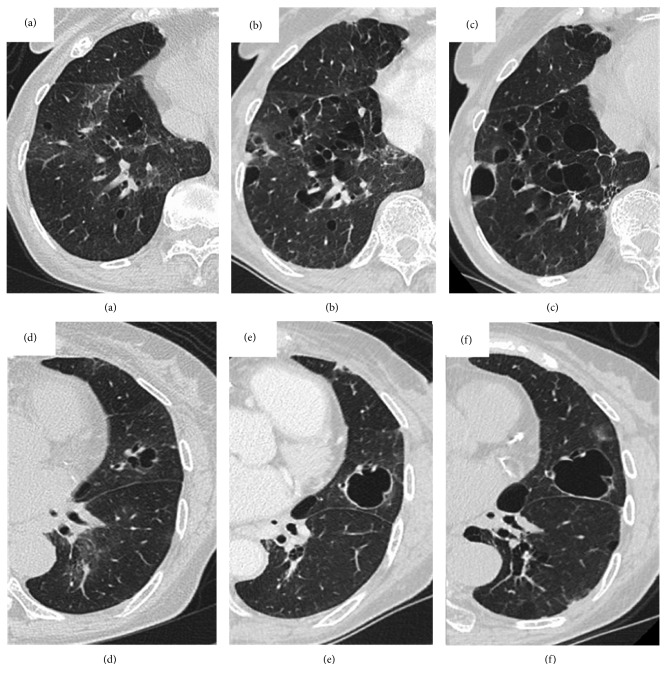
Surveillance chest computed tomography in a 72-year-old woman with Sjögren syndrome and pulmonary amyloidosis after 6 years of follow-up. The cystic lung lesions gradually increased in size and number after 6 years. (a–c) The right lower lobe and (d–f) the left upper and lower lobes are shown. (a) and (d) are images taken upon the time of diagnosis, (b) and (e) are images taken after 3 years, and (c) and (f) are images taken after 6 years.
